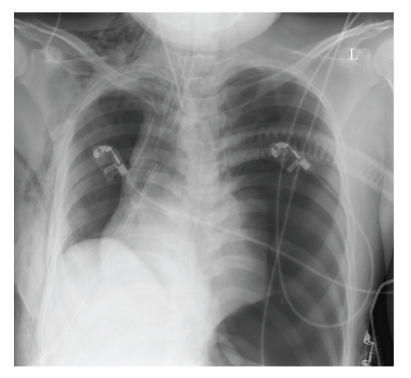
Chest X-rays of the patient showing a tension pneumothorax on the left side with mediastinal shift.
